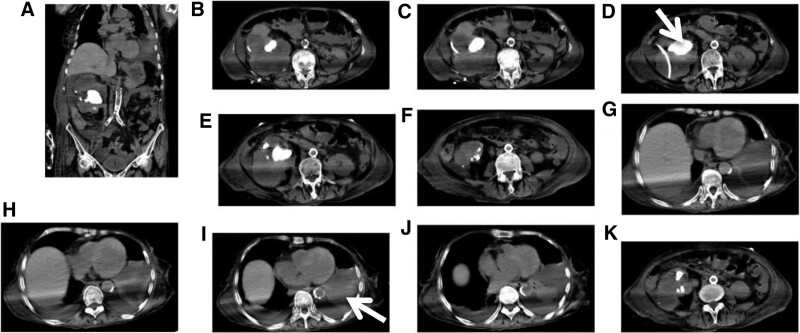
Preoperative computed tomography (CT) images. Preoperative CT imaging showed bilateral pleural effusion, bilateral interstitial pneumonia, multiple kidney stones, and severe right hydronephrosis in the patient. The arrow in the upper panel D indicated kidney stone and pigtail tube drainage. The arrow in the lower panel I denoted lung consolidation and pleural effusion.
